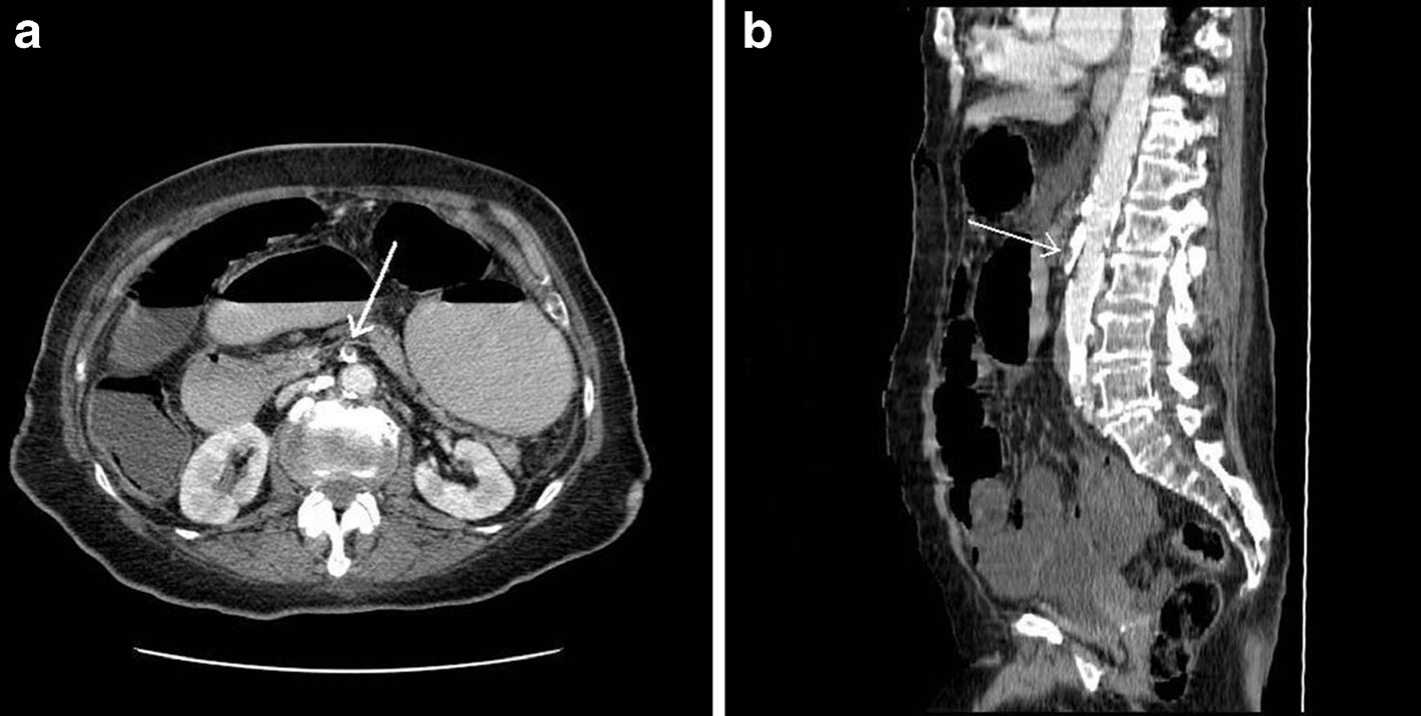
(a) Axial and (b) parasagittal images from a computed tomography scan of the abdomen demonstrating a heavily calcified aorta and superior mesenteric artery. No intravenous contrast can be seen within the superior mesenteric artery (arrow), suggesting total or subtotal occlusion.
